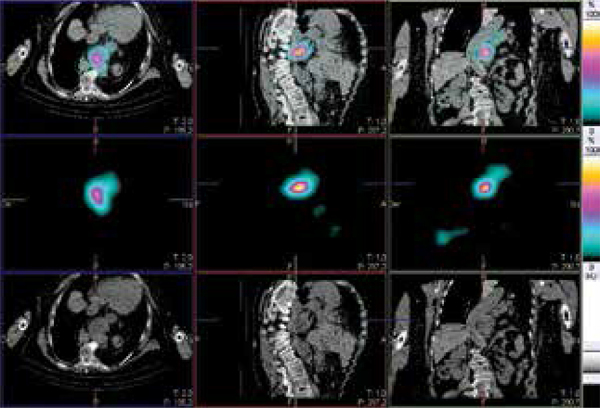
Axial, sagittal and coronal views (left, center and right columns) of hydrib iodine-131 SPECT/CT. Fused images are exposed in the upper row, the SPECT alone in the center row and CT alone in the lower row. 88 x 60 mm (300 x 300 DPI).
